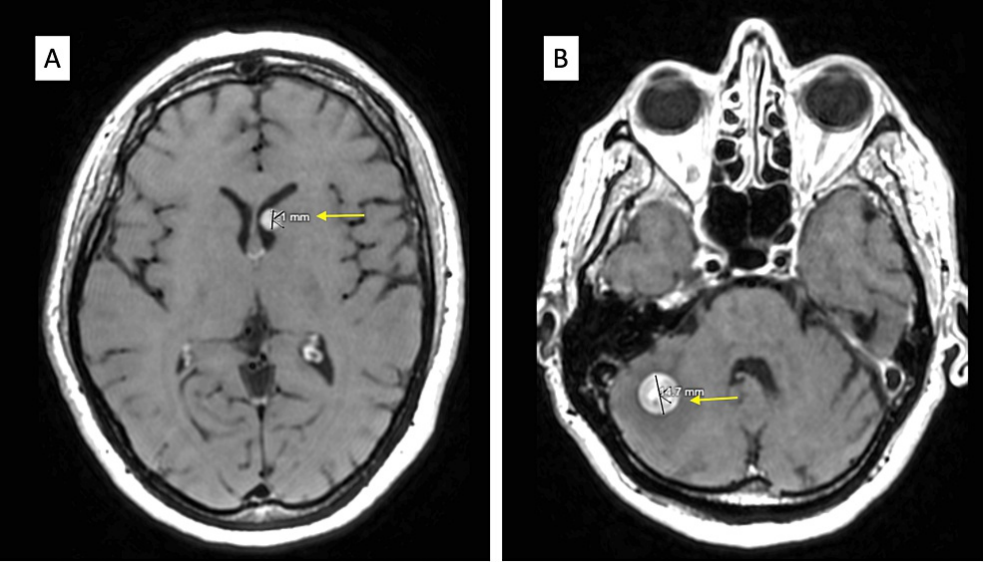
A) May 2023 MRI brain with a yellow arrow pointing to left thalamic metastasis. B) May 2023 MRI brain with a yellow arrow pointing to right cerebellar metastasis.
